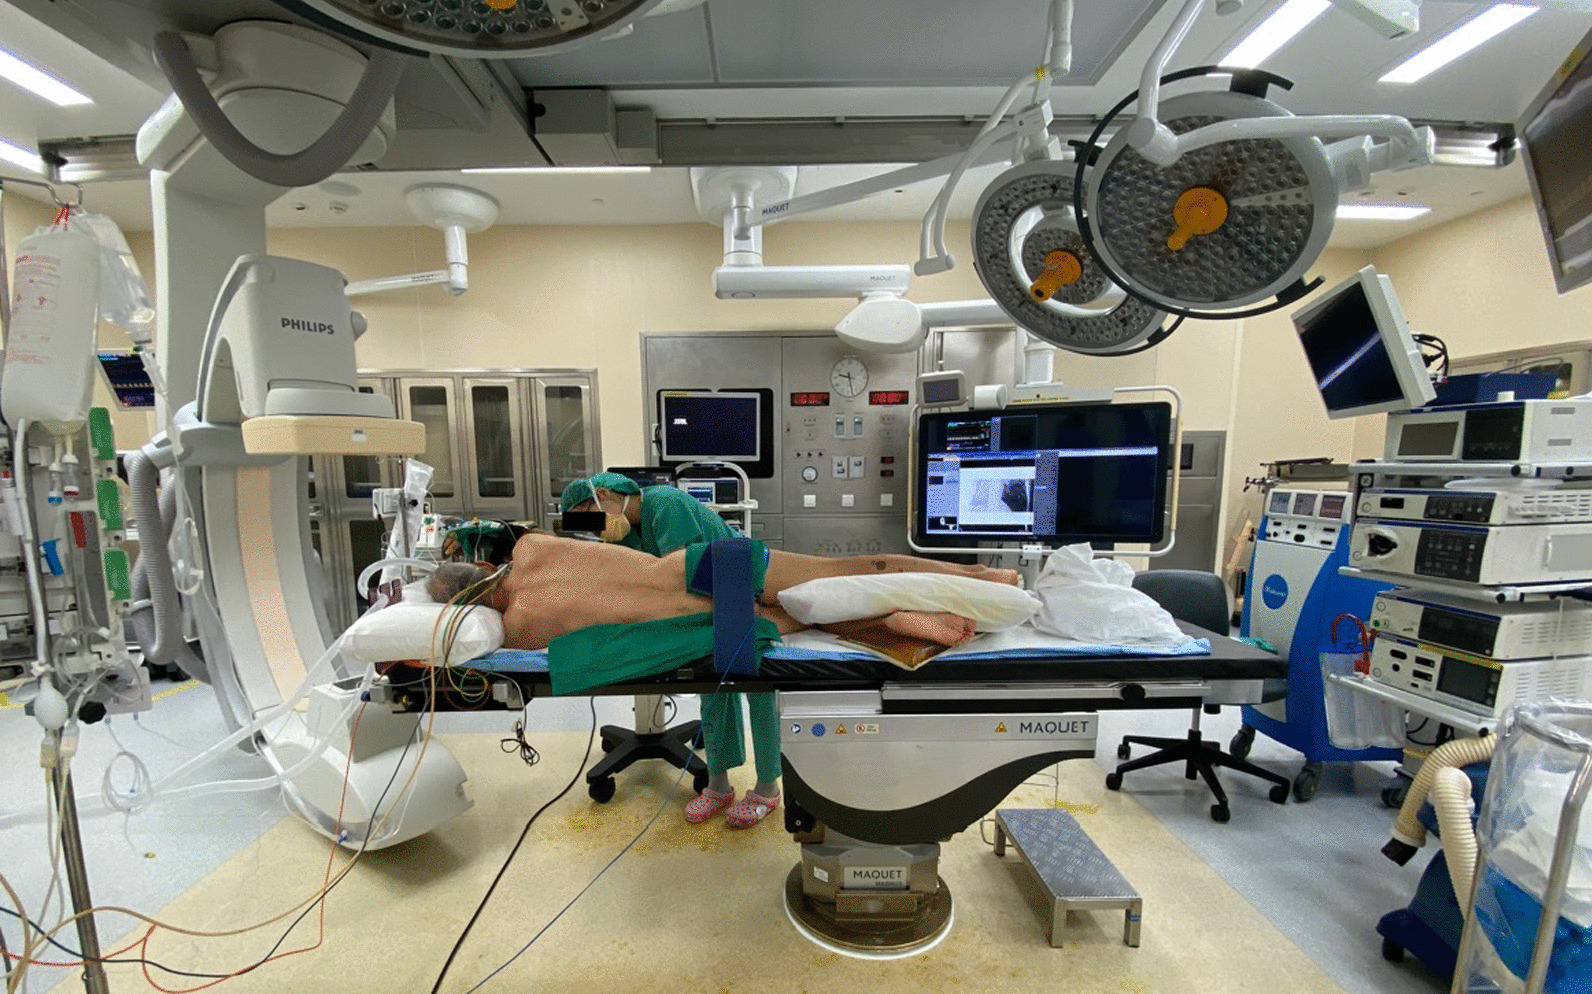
Hybrid operating room equipped with ceiling mounted Allura FlexMove fluoroscopy unit showing restricted movement of the c-arm due to patient positioning with arm in the lateral positionLesions located within 10 mm of 1st or 2nd order pulmonary arteries, as the higher CT resolution in the IR suite is required for better visualization of the vessels, to facilitate precise needle manipulation to avoid major vascular injury (Fig. 3A and B).
